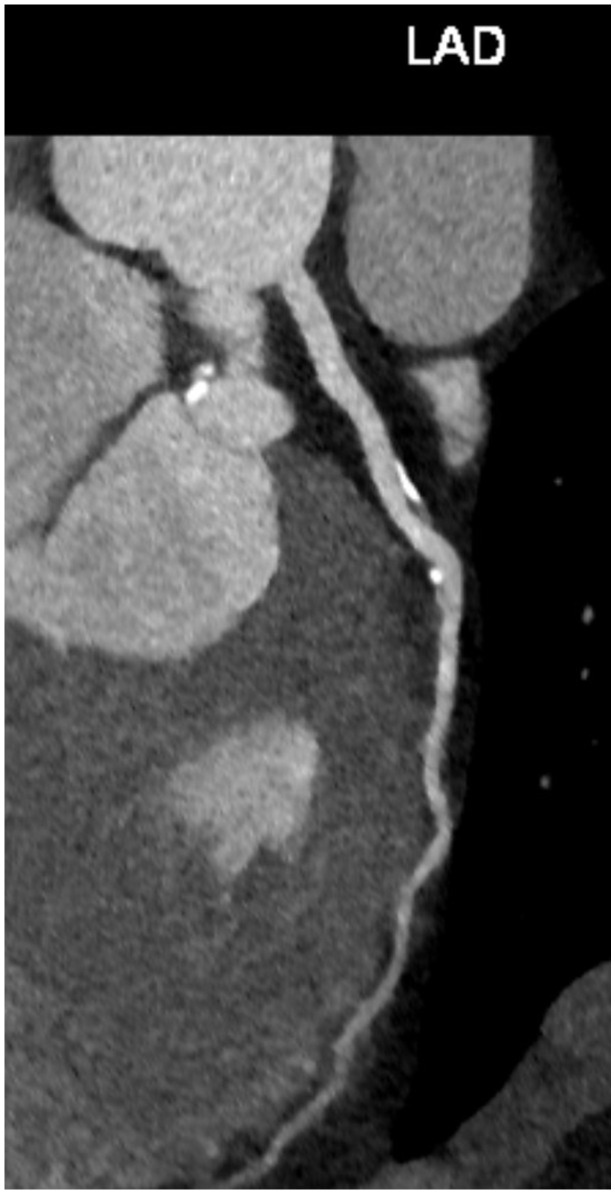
An incidental finding of left anterior descending (LAD) coronary artery plaques on a CT angiography performed in a 44-year-old male patient with a bicuspid aortic valve, dilated ascending aorta, and moderate aortic regurgitation.
